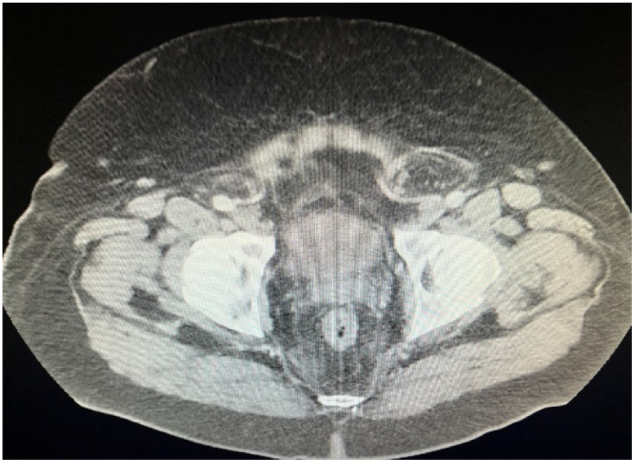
Axial image CT scan revealing recurrent bilateral fat filled inguinal hernias.
